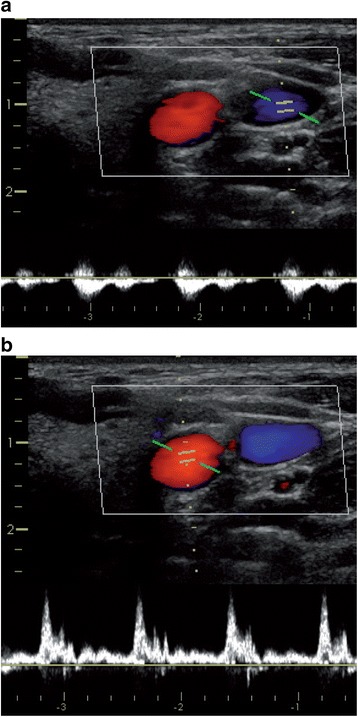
Color Doppler imaging and Doppler flow measurements. Short-axis (transverse) view of the right internal jugular vein (blue) and the carotid artery (red) using color Doppler imaging and Doppler flow measurements of the venous (a) and arterial (b) blood flow profile (Color figure online)
